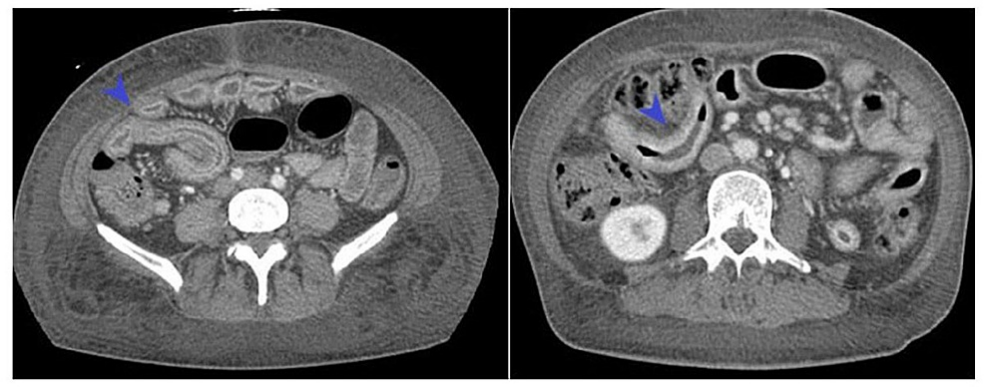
CT abdomen and pelvis, demonstrating diffuse small bowel wall thickening from the distal jejunum to TI, along with mesenteric adenopathy. TI, terminal ileum
